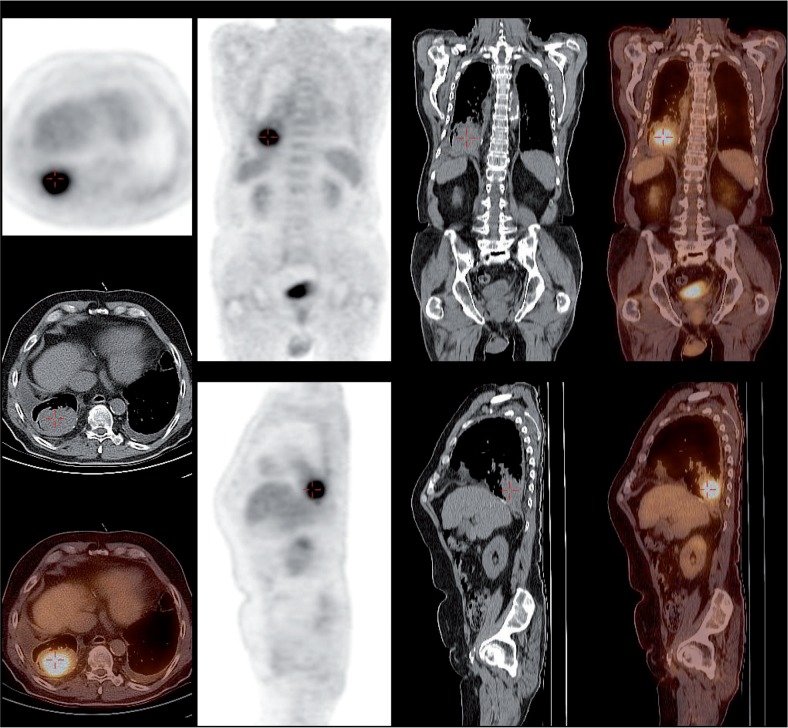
Differences in lung tumour and atelectasis seen on PET-CT.
